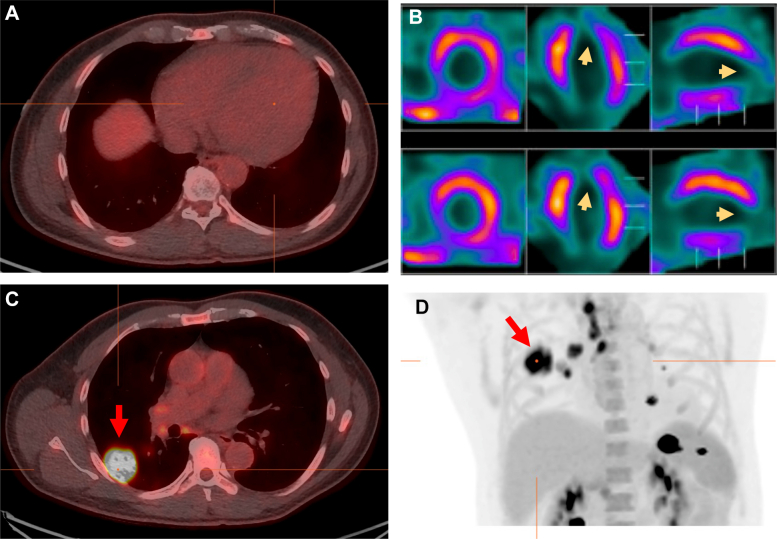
Cardiac FDG PET/CT Images (A and D) Cardiac FDG PET/CT after a high-fat low-carbohydrate diet showed no focal myocardial uptake. (B) There was focal myocardial scar involving the apex and the apical lateral walls (yellow arrows). (C and D) Intensely FDG-avid right lower lobe subpleural consolidation, likely representing an infectious etiology, was observed (red arrow). FDG = fluorodeoxyglucose; PET/CT = positron emission tomography/computed tomography.
